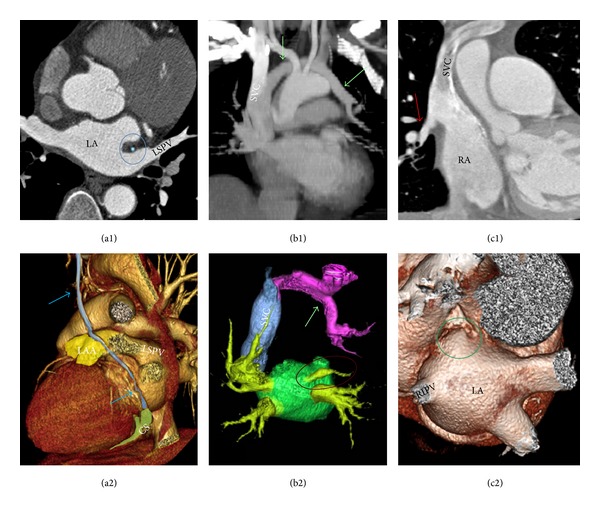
CT slices (top) and view of volume rendered CT angiographies (bottom) are shown. (a1) and (a2) demonstrate a patent vein of Marshall (blue arrow and within the blue ring) passing between the left atrial appendage (LAA) and the left superior pulmonary vein (LSPV) before joining the coronary sinus (CS). (b1) and (b2) are partial anomalous pulmonary venous (green arrows) return draining part of the left upper lung into the superior vena cava (SVC). Note that the left superior pulmonary vein exists (within red circle) but appears diminutive compared to the other veins. (c1) and (c2) show partial anomalous pulmonary venous (red arrow) return draining part of the right upper lung into the SVC. In this case, the right superior pulmonary vein is absent (green circle). RIPV = right inferior pulmonary vein. (b2) and (c2) are flipped for better orientation.
